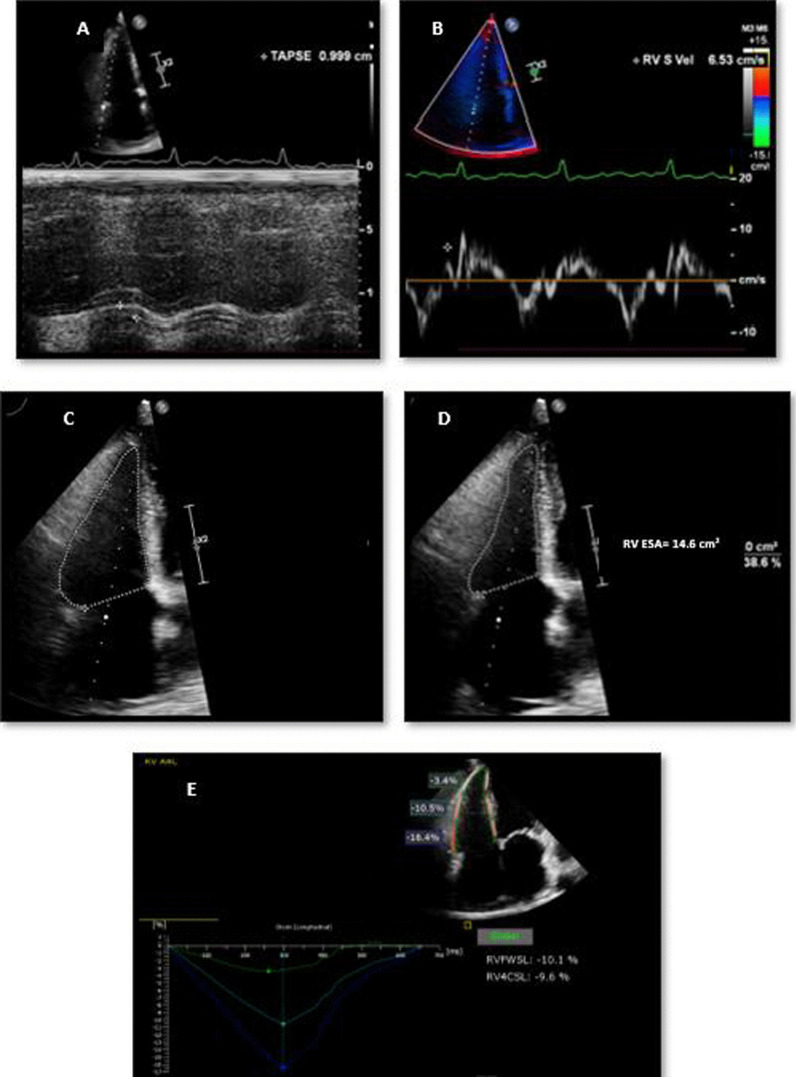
Methods of measurements of right ventricular (RV) systolic function. RV function was impaired by A tricuspid annular plane systolic excursion (TAPSE≈ 1 cm), B peak tricuspid annular systolic velocity by Pulsed-wave Tissue Doppler Imaging (S'≈ 6.5 cm) C & D RV fractional area change (FAC = 32%) calculated from RV end-diastolic area (RV EDA) and RV end-systolic area (RV ESA) and by E longitudinal RV free wall longitudinal strain (RV FWS = − 10.1%) and right ventricular global longitudinal strain (RV GLS = − 9.6%)
